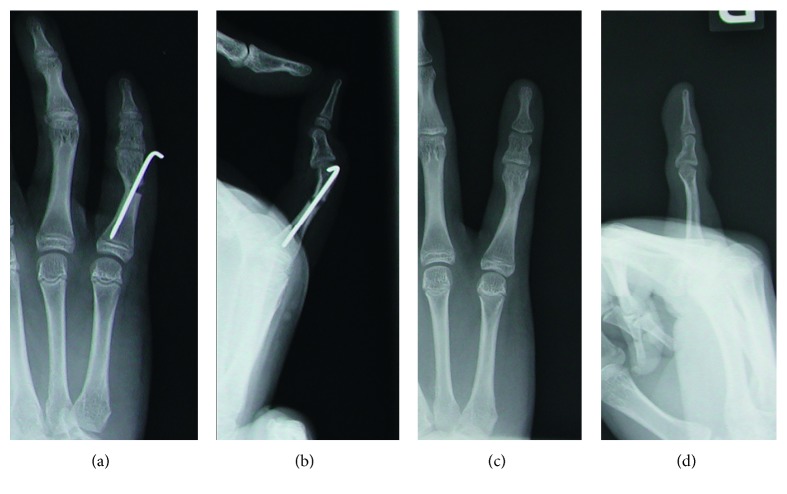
Postoperative radiographs of Case 1. (a, b) Images taken immediately after the surgery. (c, d) Images taken 1 year postoperatively.
